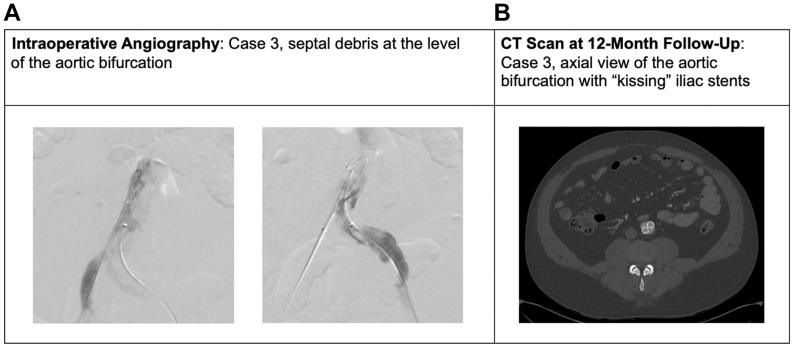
(A, B) Case 3. A 44-year-old man with hypertension and Society for Vascular Surgery/Society of Thoracic Surgeons (SVS/STS) type B_2,11L_, septal debris at the aortic bifurcation and kissing iliac stents. *CT*, Computed tomography.
